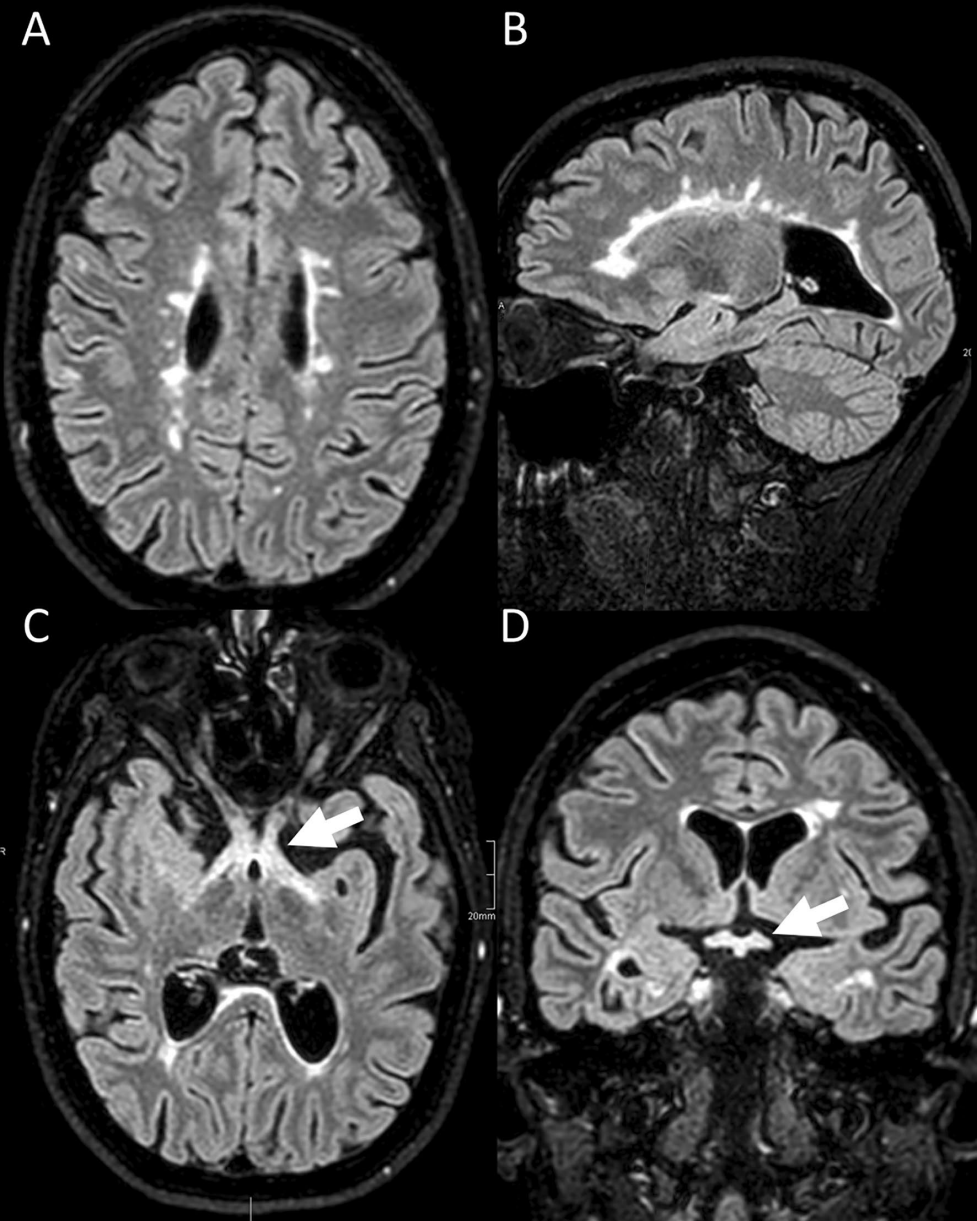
Cranial MRI (1.5T, FLAIR images) shows an acute inflammatory chiasm lesion (suggestive for NMOSD) in this patient with typical MS lesions. There were numerous chronic periventricular Dawson finger-shaped and ovoid demyelination lesions on axial (A) and sagittal (B) fluid-attenuated inversion recovery (FLAIR) images compatible with MS and an increased signal intensity in the chiasm (white arrow) and part of the adjacent optic nerves and optic tract on axial (C) and coronal (D, white arrow) images
